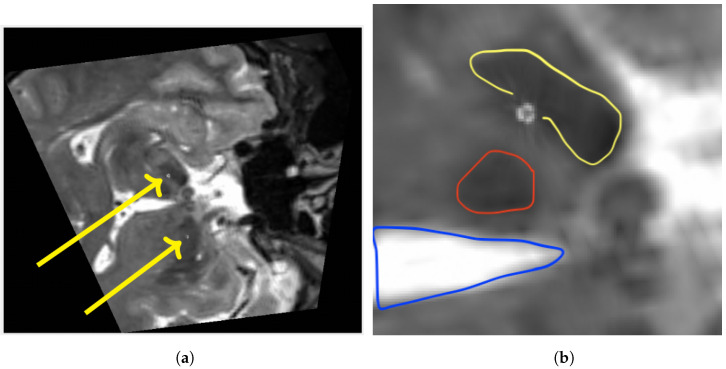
Fused image after multiplanar reformation (MPR): The images are displayed in accordance with scientific convention. Preoperative T2-weighted MRI (a), and cutout view of the same slice (b). Characteristic hypointensities depicting both the right subthalamic (yellow) and red nucleus (red) as well as the third ventricle (blue) on preoperative T2-weighted MRI. Each segmented contact point can be identified within the anatomical target area.
